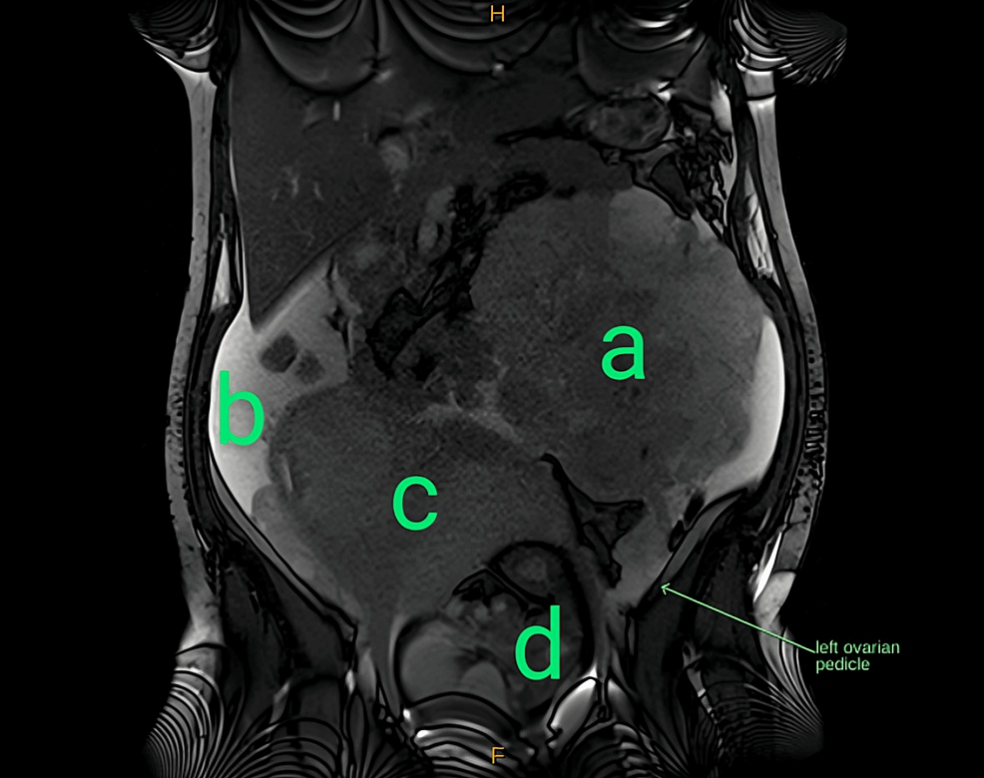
MRI T2 coronal plane showing the left ovarian pedicle (arrow) with insertion into the complex left upper abdominal mass (a), consistent with an ovarian origin; moderate ascites can also be appreciated (b). Uterine displacement (c) and the preterm fetus are also shown (d).
